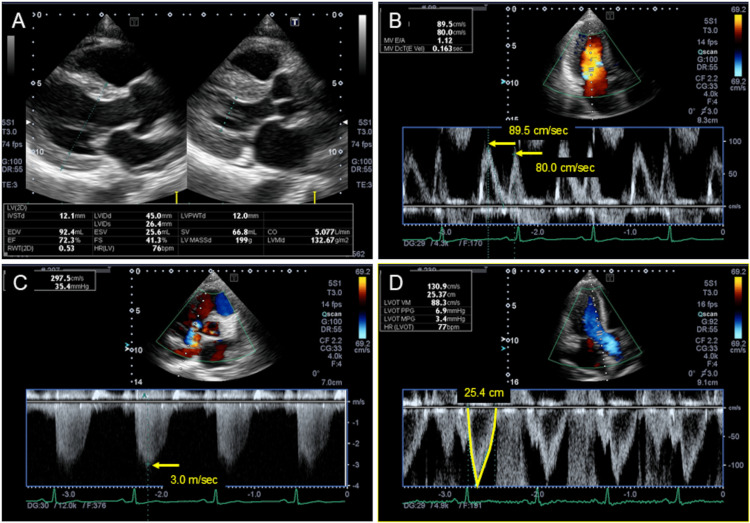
(A) A transthoracic echocardiogram revealed LV hyperkinesia with an ejection fraction of 72%. Doppler echocardiographic measurements disclosed the following values: (B) early-to-late mitral peak flow velocity ratio, 1.1; (C) peak tricuspid regurgitation velocity, 3.0 m/second; and (D) LV outflow tract velocity time integral, 25.4 cm LV: left ventricular
